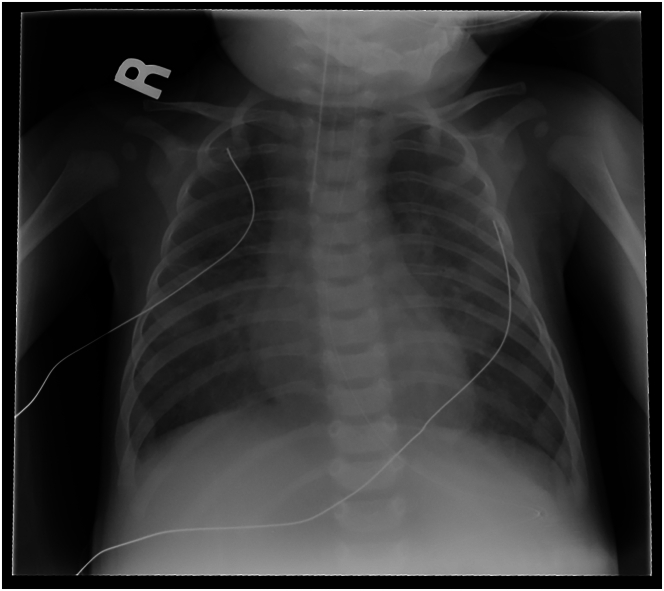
Chest radiograph of a 3-month-old child presenting with respiratory failure secondary to multivessel pulmonary vein stenosis. The image demonstrates a bilateral pattern of interstitial opacities as a result of pulmonary venous hypertension and interstitial edema. R, right.
